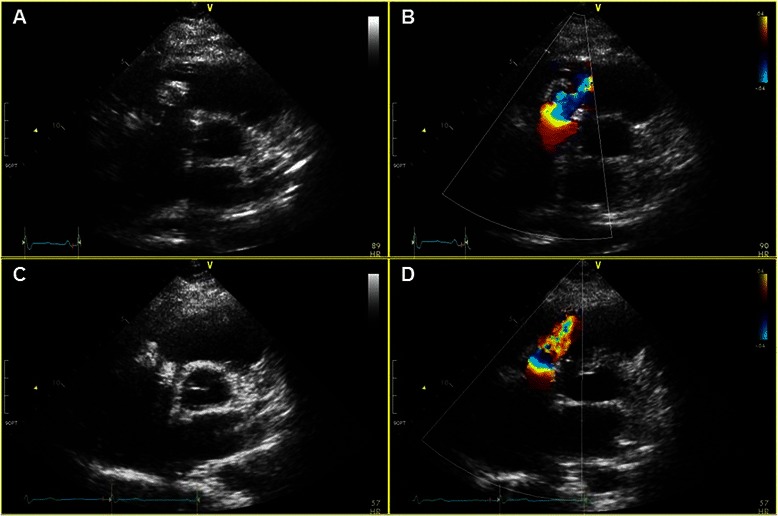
Echocardiograms performed before the patient’s final discharge (a and b) and 8 months after surgery (c and d). Both showed a well-functioning aortic valve homograft in the tricuspid position
